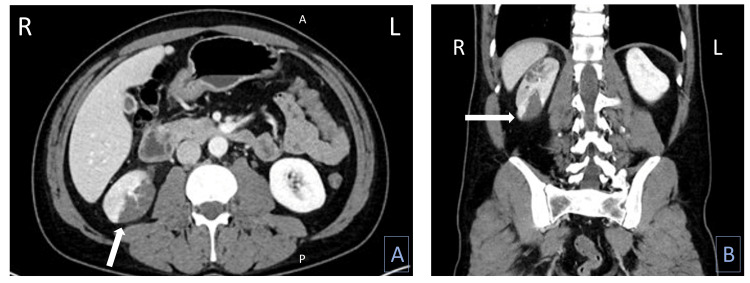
CT of abdomen/pelvis with IV contrast showing right upper and lower pole renal infarcts (arrow), (A) axial view and (B) coronal view. A: anterior; P: posterior; R: right; L: left
